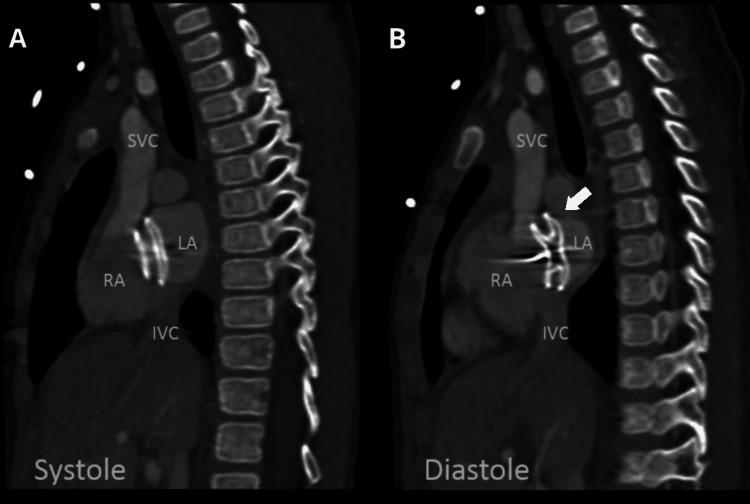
Cardiac CT angiography in the sagittal oblique (bi-caval) projection Both discs of the ASD device within the atrium in systole (A). However, in diastole (B), there is significant superior displacement of the left atrial disc into the roof of the left atrium (arrow) RA: right atrium; RV: right ventricle; LA: left atrium; SVC: superior vena cava; IVC: inferior vena cava; ASD: atrial septal defect
